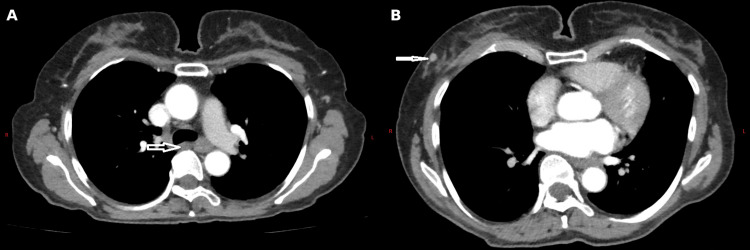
Contrast-enhanced computed tomography images showing (A) a paraoesophageal lymph node and (B) right axillary lymphadenopathy.
